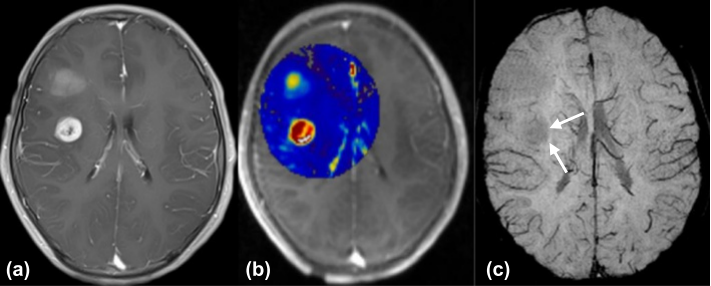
Images of a 13-year-old boy with left frontal glioblastoma. (a) The contrast-enhanced axial T1-weighted image shows a mass with regular peripheral rim enhancement. (b) The high K^trans^ values within the tumor indicates a high permeability of microvessels. (c) However, SWI reveals no evidence of ITSS (arrows).
